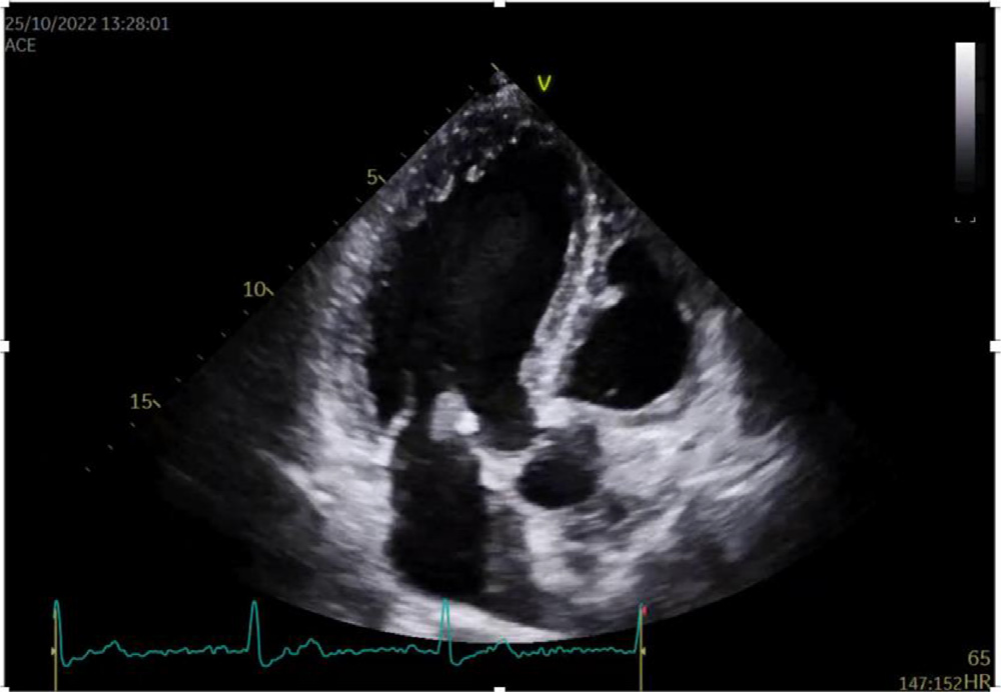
2D Transthoracic echocardiography image in the PLAX view showingg a globular mass attached to the mitral valve.
